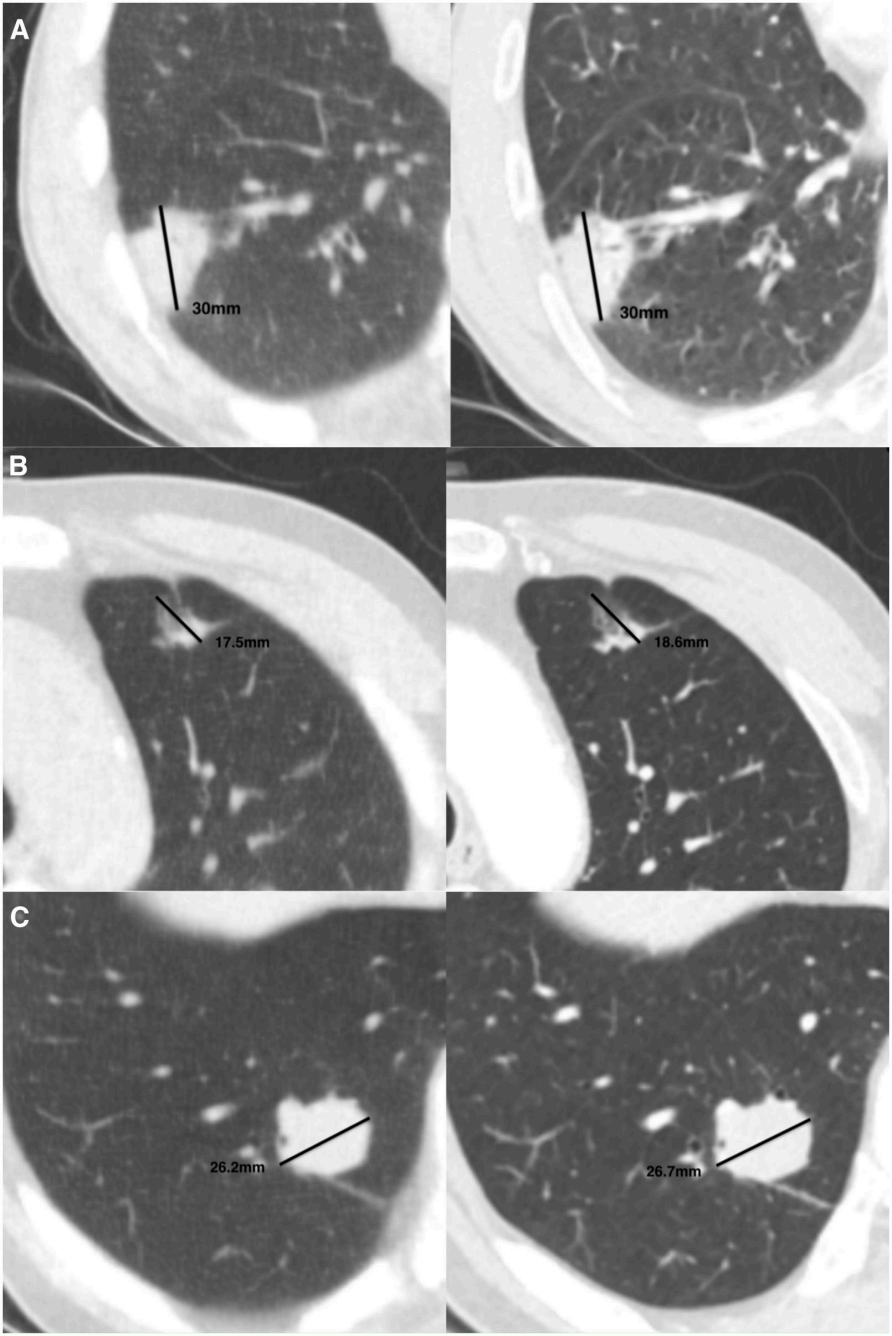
Representative cases. Comparison of ULDCT images (left) and diagnostic contrast-enhanced CT images (right). The first two ULDCT scans (A and B) had a radiation dose of 0.07 mSv (70 kV/20 mA). The third ULDCT scan (C) had a radiation dose of 0.14 mSv (70 kV/40 mA). The mean radiation dose of the contrast-enhanced CT scans (A, B, and C) is 2.24 mSv (120 kV/100-200 mA). The dose of ULDCT is only 3%-6% of that of contrast-enhanced CT. (A) A 64-year-old male patient with a 30-mm lobulated nodule in the basal segment of the lower lobe of the right lung, as detected and measured by ULDCT and contrast-enhanced CT, which was pathologically confirmed as invasive squamous carcinoma. (B) A 56-year-old male patient with chronic inflammation of the bronchial mucosa detected by biopsy, had a part-solid nodule in the apical posterior segment of the upper lobe of the left lung measuring 17.5 mm by ULDCT and 18.6 mm by contrast-enhanced CT. The morphology of this nodule is irregular with bronchogram. (C) A 57-year-old man with a lobulated nodule in the basal segment of the lower lobe of the right lung, measuring 26.2 mm by ULDCT and 26.7 mm by contrast-enhanced CT, was pathologically confirmed as invasive adenocarcinoma. Although the malignant nodules detected by ULDCT appear to be ill-defined compared with diagnostic contrast-enhanced CT, the shape of these nodules could be recognized and their size could be measured. (These cases are from the author's institute.)
